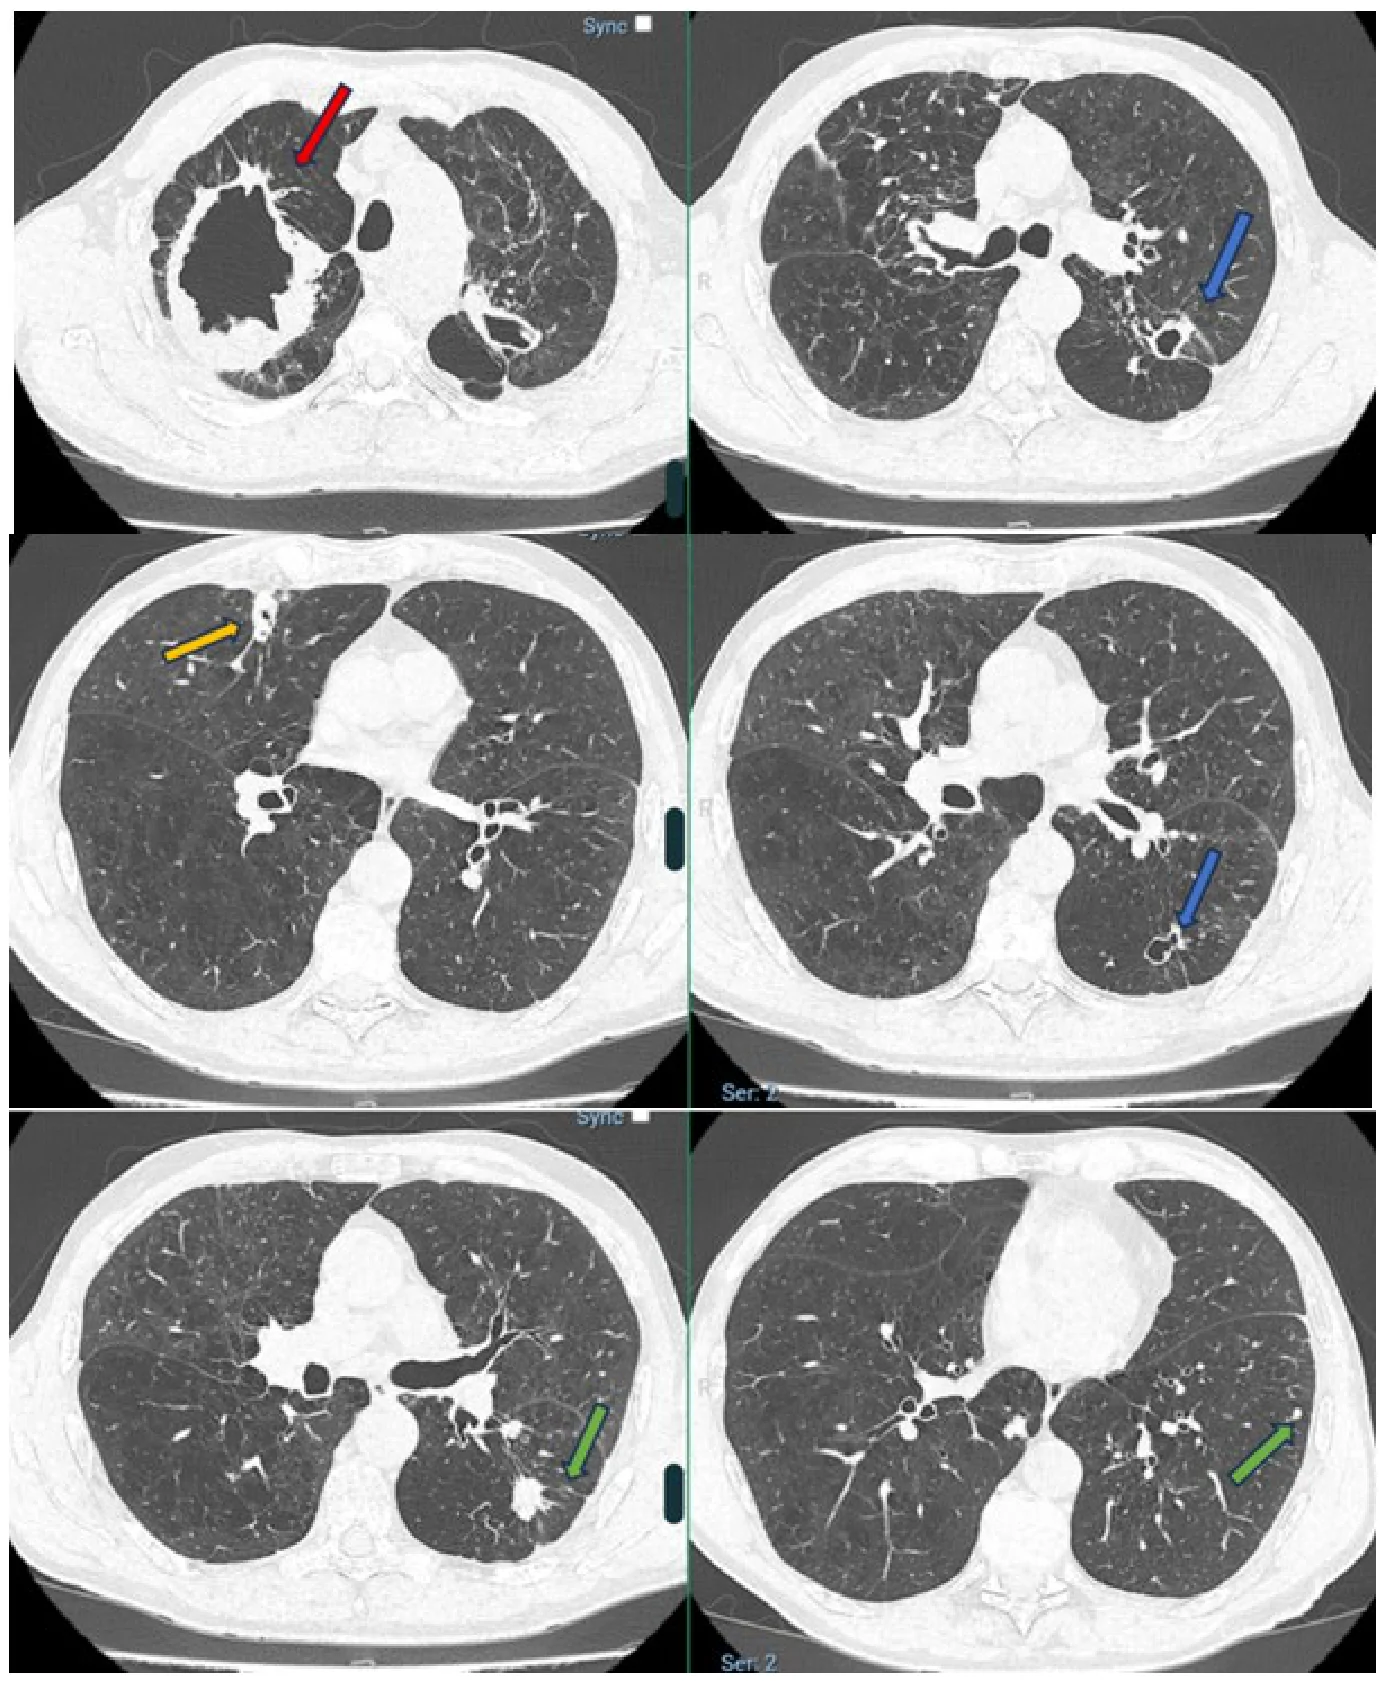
Thoracic CT scan showing a 9.7 × 7.4 cm thick-walled lesion with an irregular internal contour and non–iodine-enhancing areas, containing aerated components and adjacent interstitial thickening, located in the apical and posterior segments of the right upper lobe (red arrow). Inter-connected cavitary lesions are also seen in the apicoposterior and apical segments of the left upper lobe (blue arrows), featuring a draining bronchus and thickened walls, the largest measuring 3 × 2.5 cm. Diffuse pulmonary nodules are present, some calcified and others displaying a “tree-in-bud” pattern, particularly in the left lower lobe (green arrows). A newly developed pseudonodular pulmonary consolidation with adjacent micronodules, slightly iodine-enhancing, is observed in the middle lobe (yellow arrow). The lung parenchyma is markedly emphysematous.
